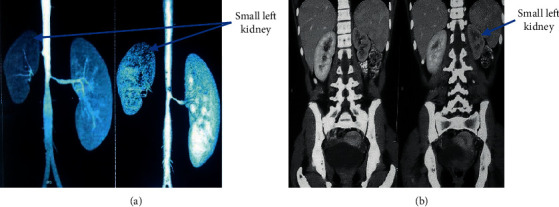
CTA showing small left kidney.
